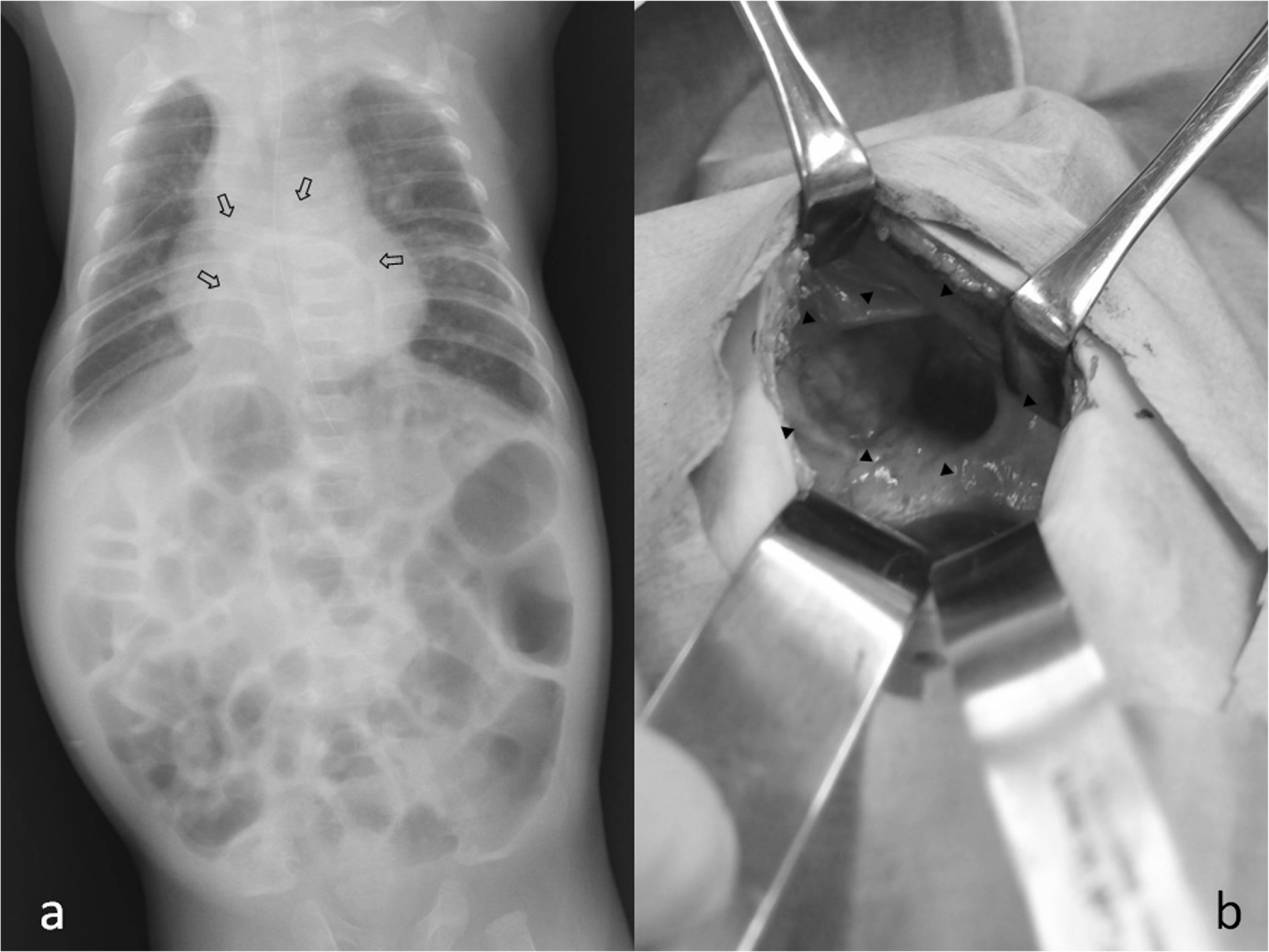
Radiograph on day 161 of life (a) and photograph during direct closure of the diaphragm (b) in Case 2. a On day 161, intestinal gas was detected in the mediastinum (➩). b A retrosternal hernial orifice (▼) was observed at operation and the defect was closed directly
